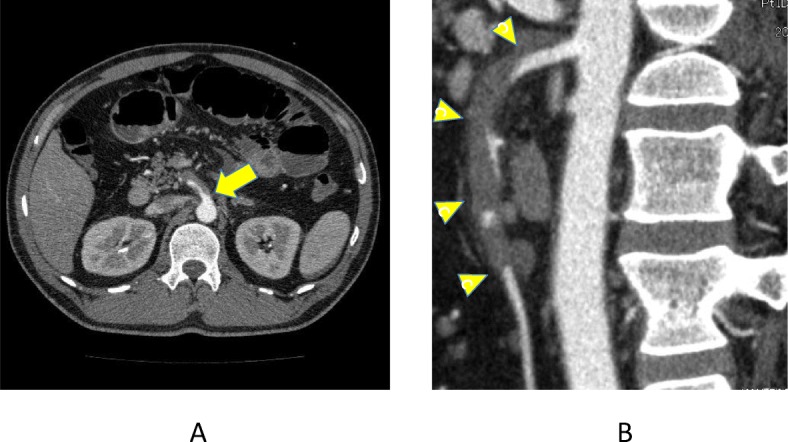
Computed tomography images 4 days after the symptom onset. a The main trunk of the superior mesenteric artery was dissected from its origin (arrow). b Sagittal multiplanar reconstruction computed tomography showed the thrombosed false lumen and compressed true lumen with thrombosis at the distal portion (arrowheads) and enhancement at the more distal portion
